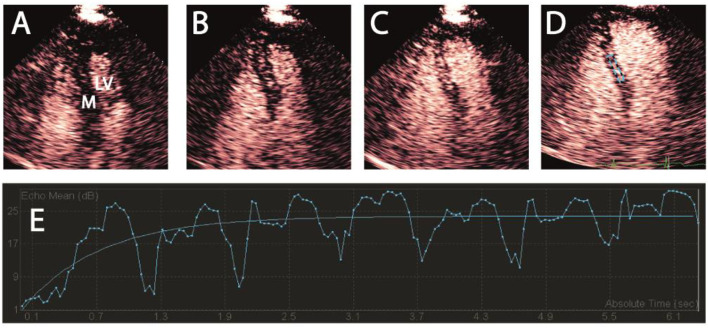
Myocardial contrast echocardiography. Contrast-enhanced transthoracic apical 4-chamber view end-systolic. After microbubble destruction with a high MI-pulse, replenishment of contrast is visible in the myocardium. (A–C) Subsequent replenishment of myocardial contrast, (D) ROI in the myocardial septum, (E) Example of myocardial replenishment in a time-video intensity curve. Fluctuations in the myocardial blood volume during replenishment, as demonstrated in this figure, is caused by the cardiac cycle of systole and diastole. MI, mechanical index; ROI, region of interest; M, myocardial; LV, left ventricle.
